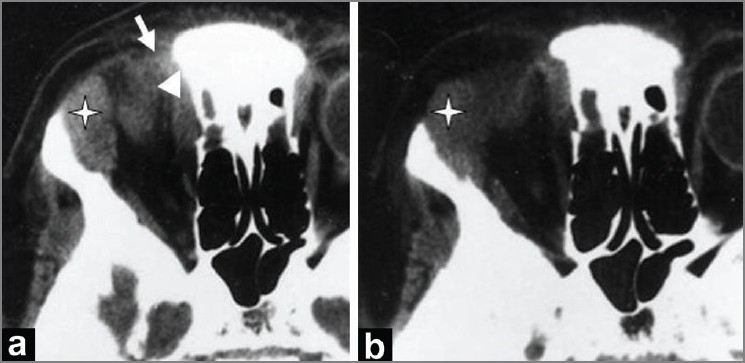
A 9-year-old girl presented with unilateral painless nonpulsatile proptosis of the right eye. Axial contrast-enhanced CT scans (a,b) of the orbit, show an enlarged lacrimal gland (asterisk), fine-needle aspiration cytology of which demonstrated tuberculous granulomas with caseation. Sight heterogenous soft tissue thickening (arrow in a) is also seen in the superomedial aspect of the orbit with slight irregularity of the adjacent bone (arrowhead in a)
